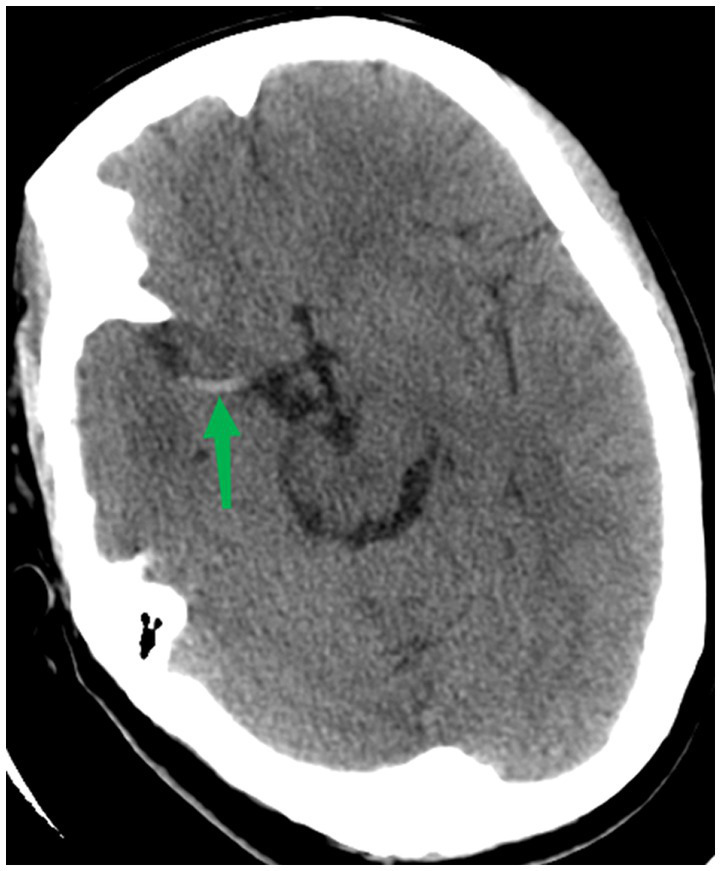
CT Scan showing a typical hyperdense middle cerebral artery sign.
